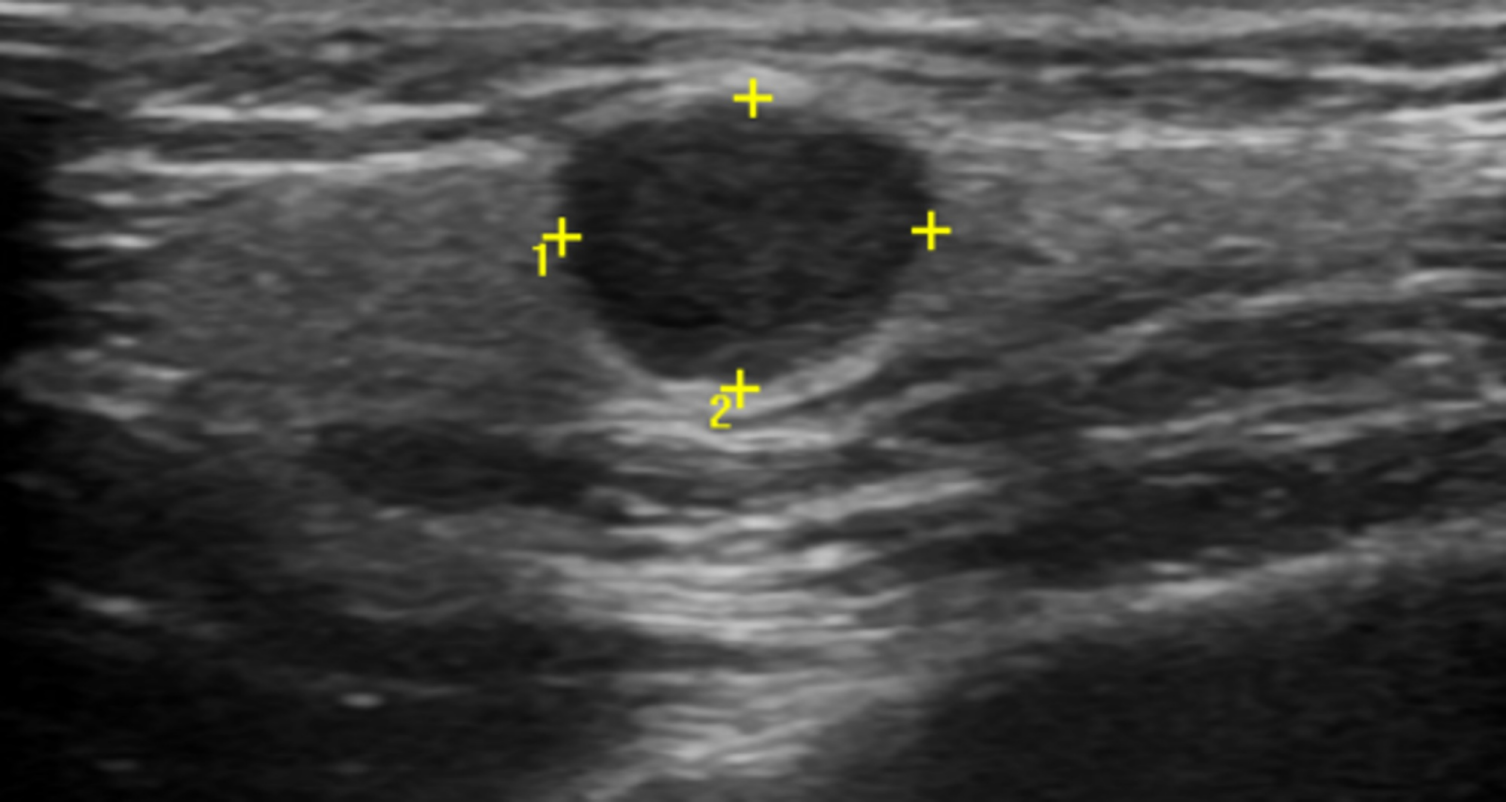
Ultrasonography showing the tumor in front of parotid gland above the masseter muscle
